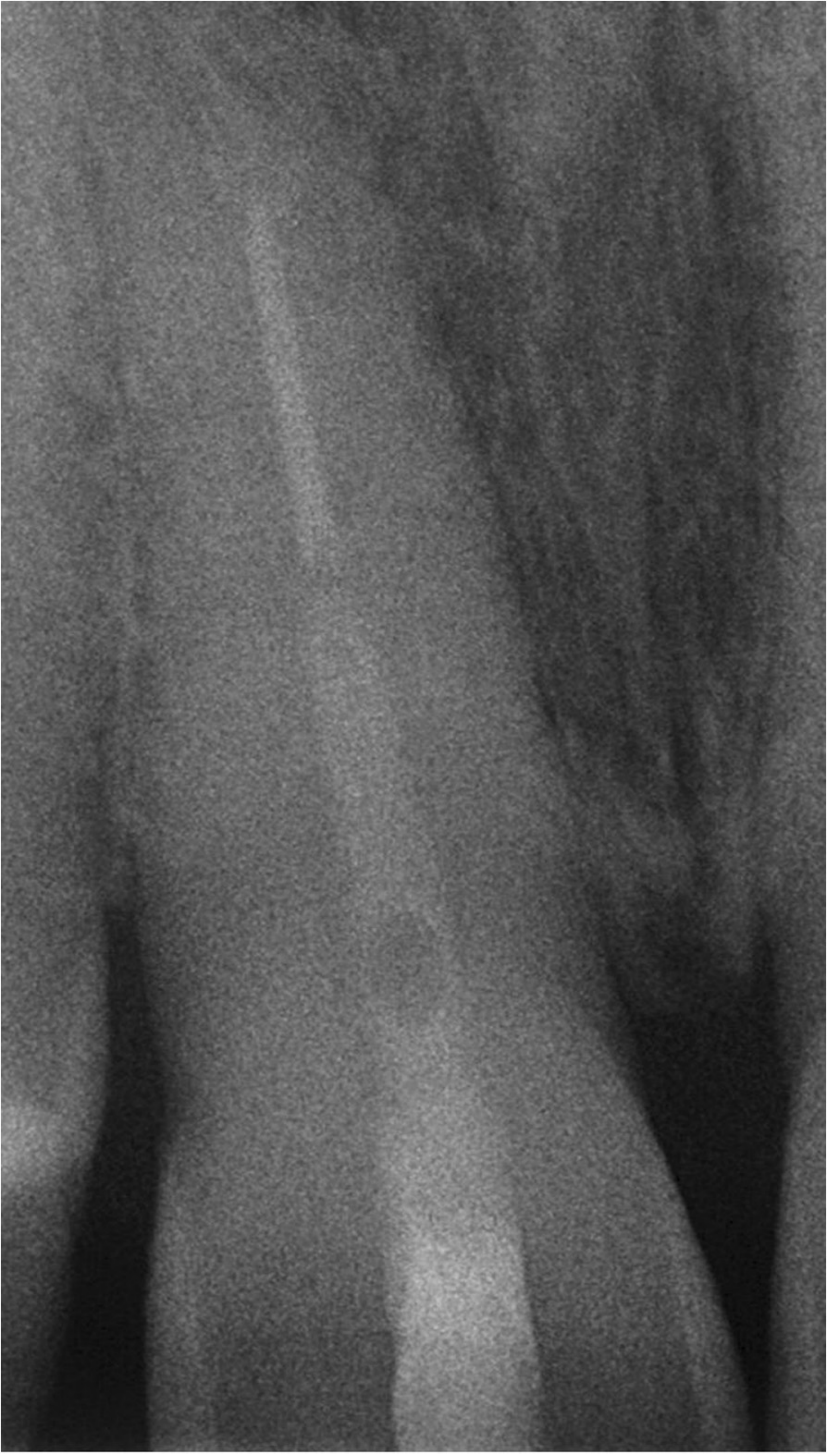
Control radiograph of tooth 11 24 months after replantation.
